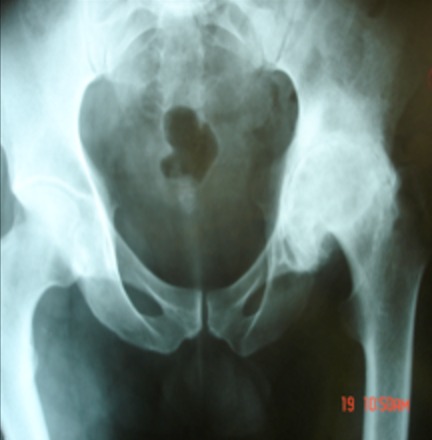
Radiographie préopératoire, montrant la destruction ostéo-cartilagineuse à gauche avec pincement globale de l‘interligne articulaire coxo-fémorale, érosion sous chondrale, déformation en forme de champignon de la tête fémorale avec ascension du grand trochanter
